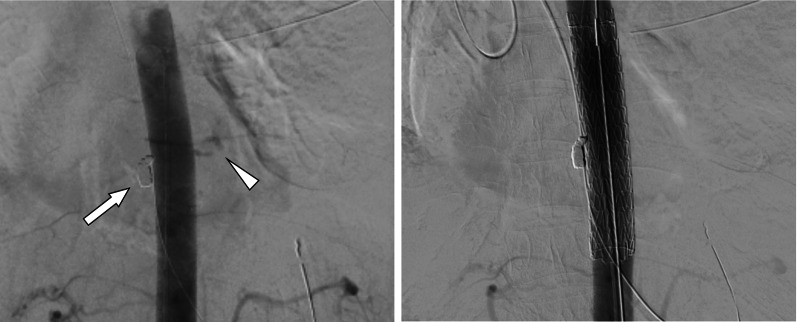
Computed tomography findings after transcatheter embolization and thoracic endovascular aortic repair. Digital subtraction angiography showing the coil placed during transcatheter arterial embolization (arrow) and extravasation of contrast medium adjacent to the descending aorta (arrowhead) (right). After deploying the thoracic aortic stent-graft, the extravasation disappeared (left)
